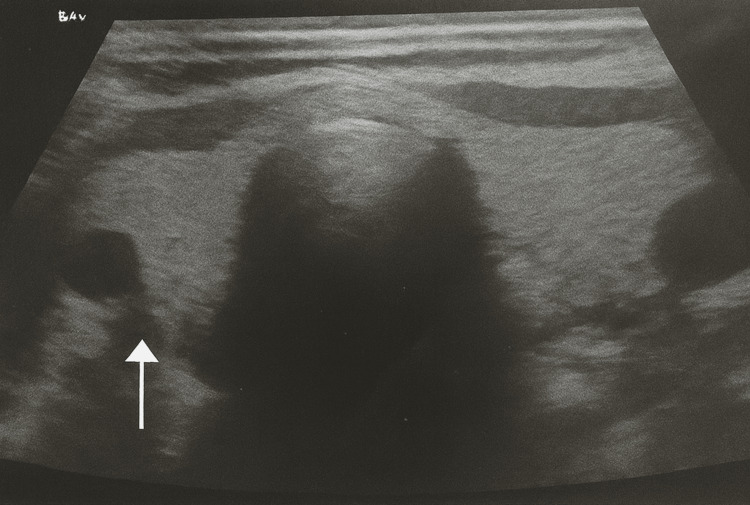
Neck ultrasound showing a 7-mm hypoechoic lesion posterior to the left thyroid lobe (white arrow), consistent with a parathyroid lesion.
